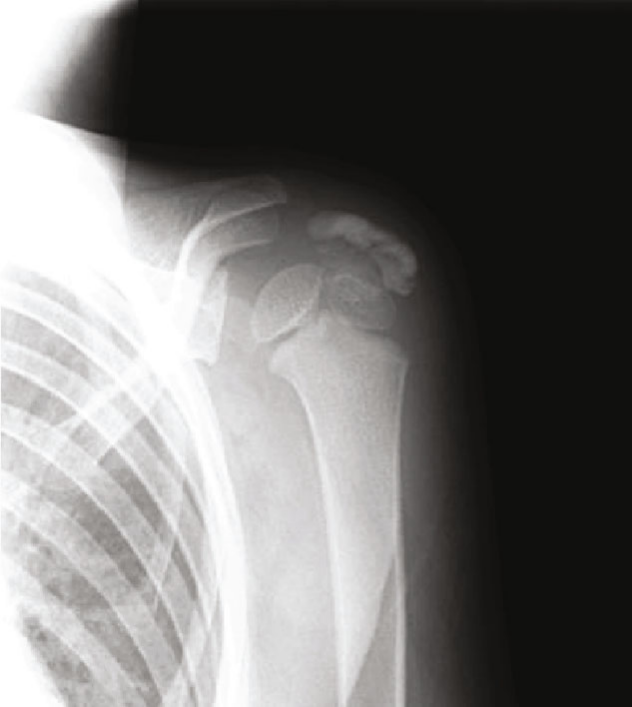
Initial radiograph of the left shoulder.
